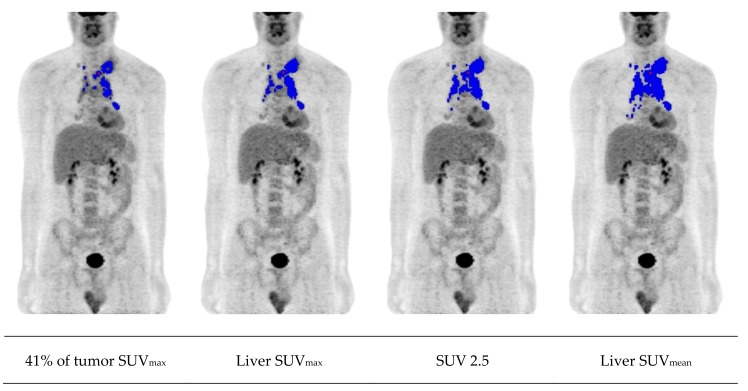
Metabolic tumor volume (MTV) measurement in a patient with advanced-stage Hodgkin lymphoma based on different thresholding methods. SUV, standardized uptake value; SUV_max_, maximum SUV; SUV_mean_, mean SUV.
